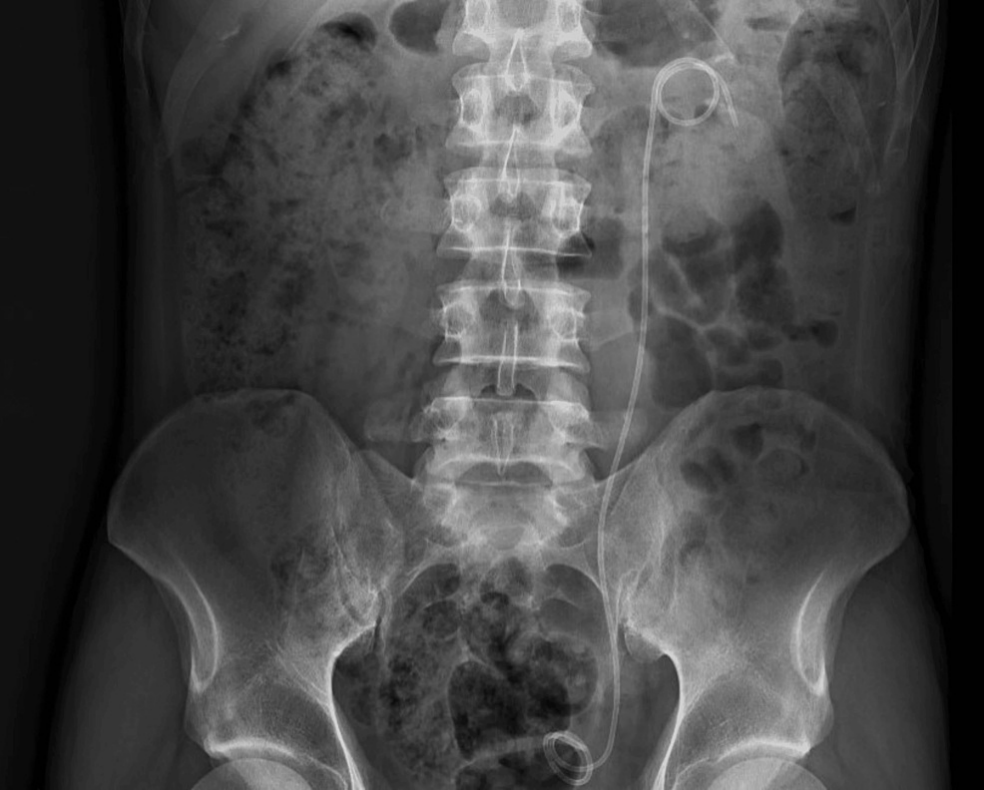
X-ray image showing new double J stent implanted on left side; ureter and kidney free of calculi.
